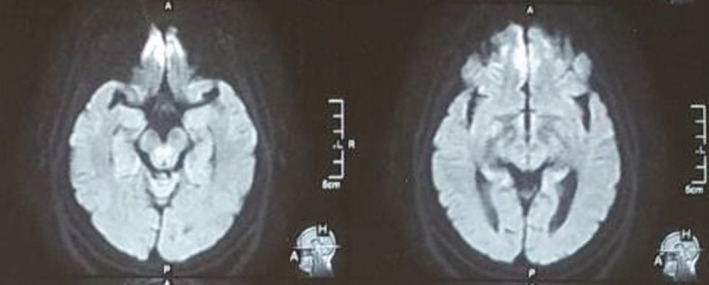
DWI high signal intensity in bilateral frontal (right> left) and occipital cortex
